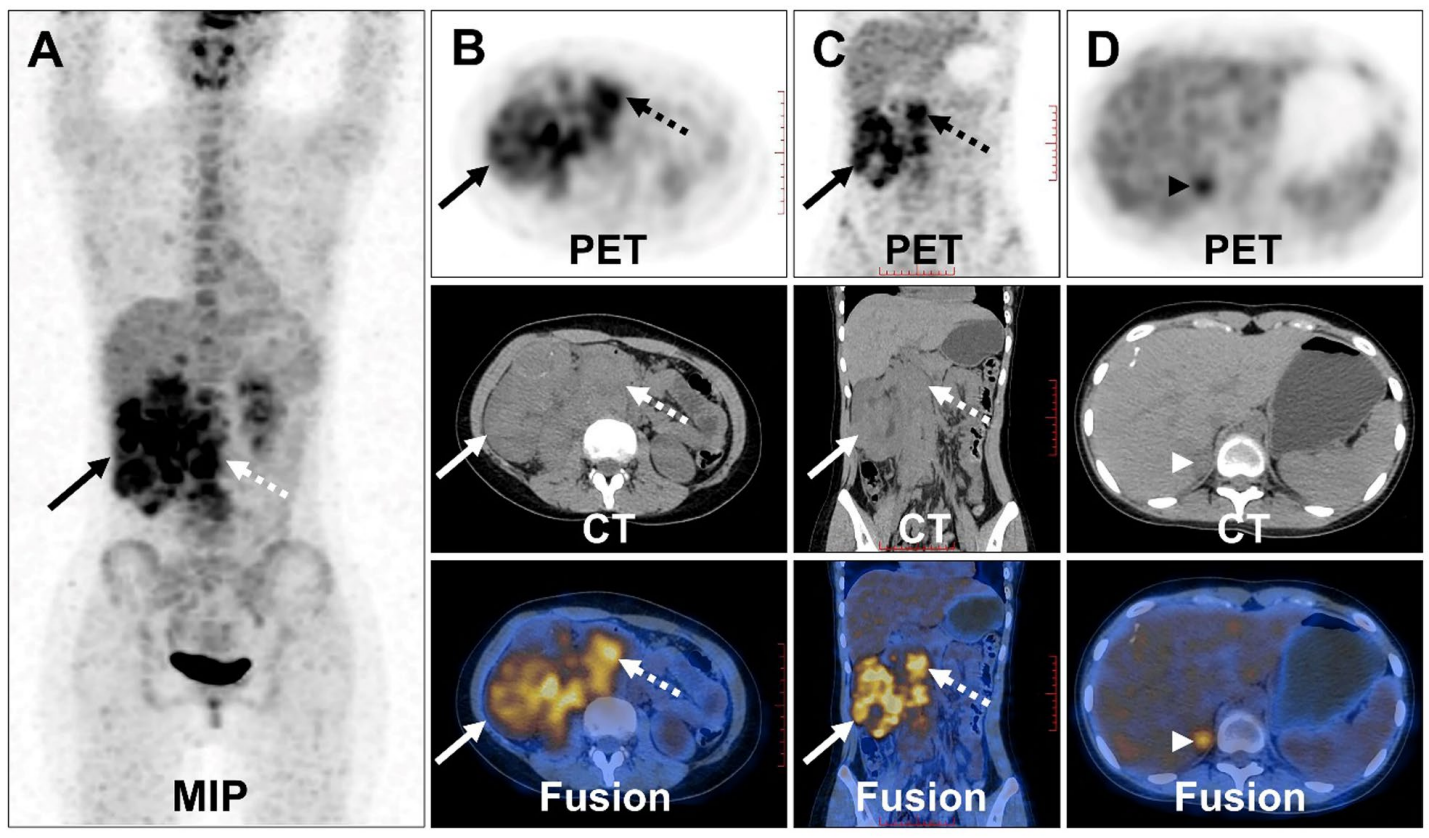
^18^F-FDG PET/CT images of Xp11.2 RCC. (A) The anteroposterior 3-dimensional maximum intensity projection image (MIP) demonstrated increased metabolic activity in the right kidney (long arrows) and retroperitoneal lymph nodes (dashed arrows). (B,C) Transverse images and coronal images showed multiple irregular occupancies in the right kidney with unclear borders, showing a heterogeneous increase in FDG uptake, with SUVmax values ranging from 2.3 to 5.2. Multiple enlarged and fused lymph nodes were observed in the medial part of the right kidney and the retroperitoneum, exhibiting a heterogeneous increase in FDG uptake, with SUVmax values ranging from 4.1 to 8.7. (D) Transverse images showed a soft tissue density nodule of the right adrenal gland measuring approximately 1.2 cm × 2.0 cm (arrowheads), with increased FDG uptake (SUVmax = 4.4).
